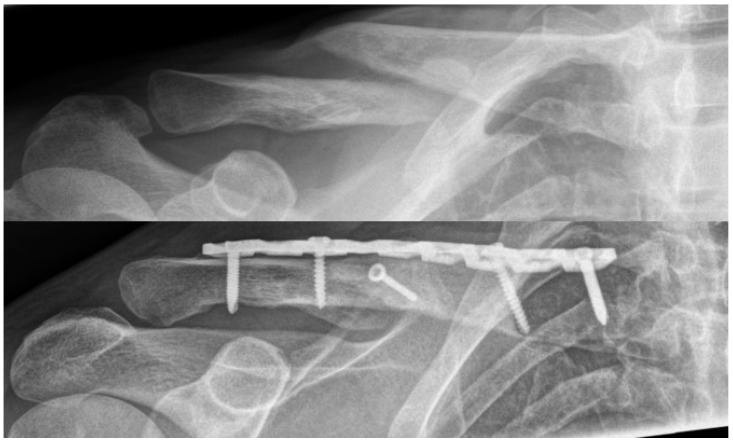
Examples of anterior–posterior radiographs of an atrophic nonunion following non-surgical treatment and following revision surgery without bone graft.
